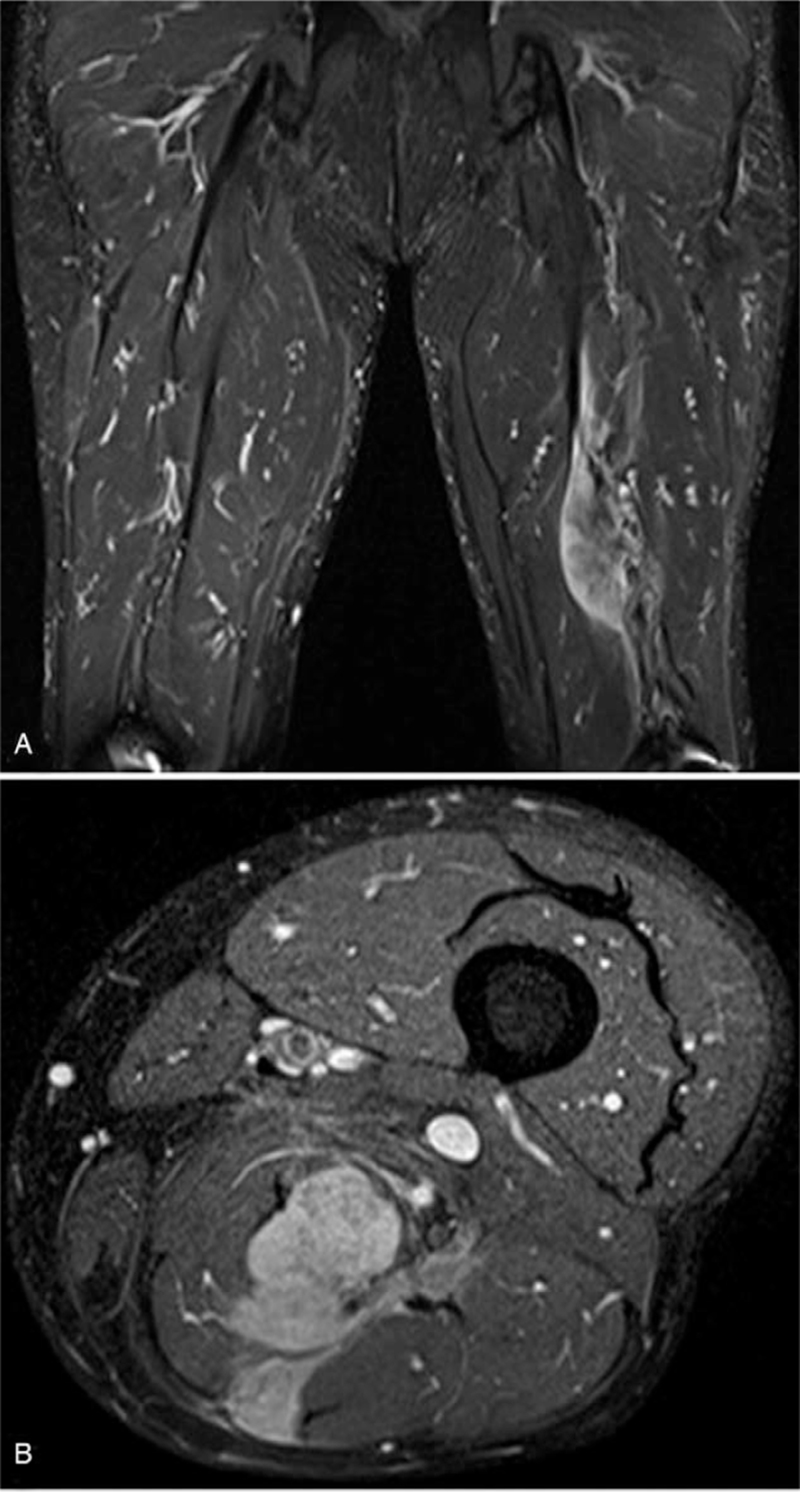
MRI showed a 12.5 × 6 × 3.5 cm sized irregular abnormal signal intensity with a vivid, enhancing soft tissue mass in the left posterior thigh, along the semimembranosus and semitendinosus muscle. MRI = magnetic resonance image.
